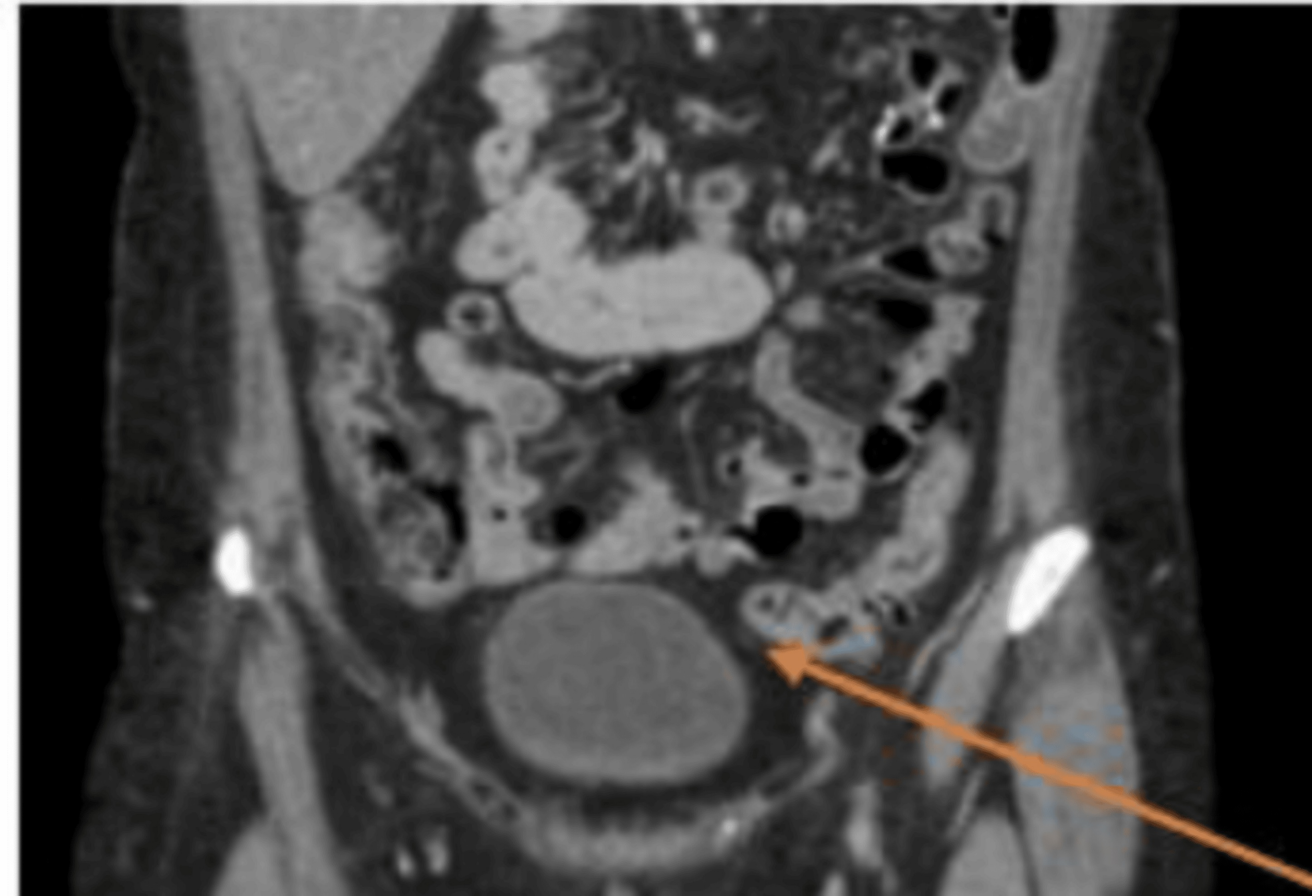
CT of the abdomen after treatment. The arrow shows the gut with no fecal retention.
